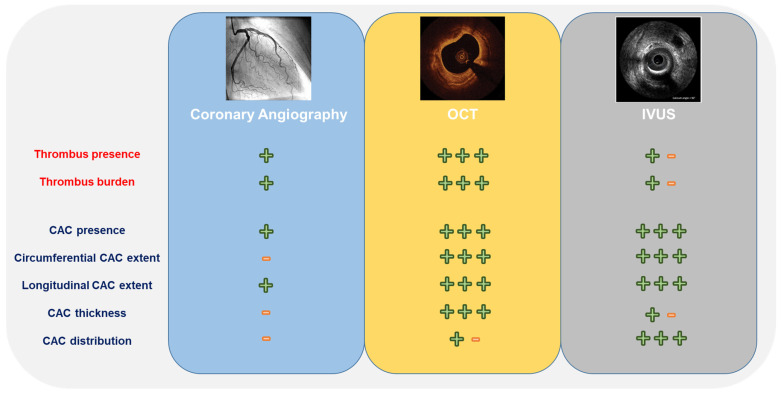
Characterization of thrombotic and heavily calcified coronary lesions by angiography and intracoronary imaging (intravascular ultrasound [IVUS] or optical coherence tomography [OCT]). Abbreviation: CAC, coronary artery calcification.
